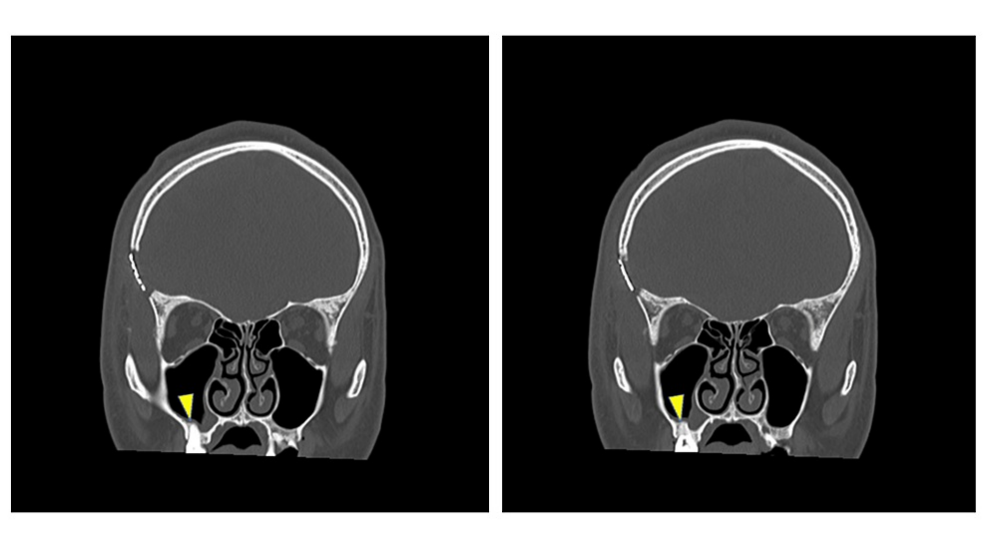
Coronal section including the upper dentition on the follow-up CT. A tooth with root inflammation showed osteolysis and was entrenched in the maxillary sinus.
